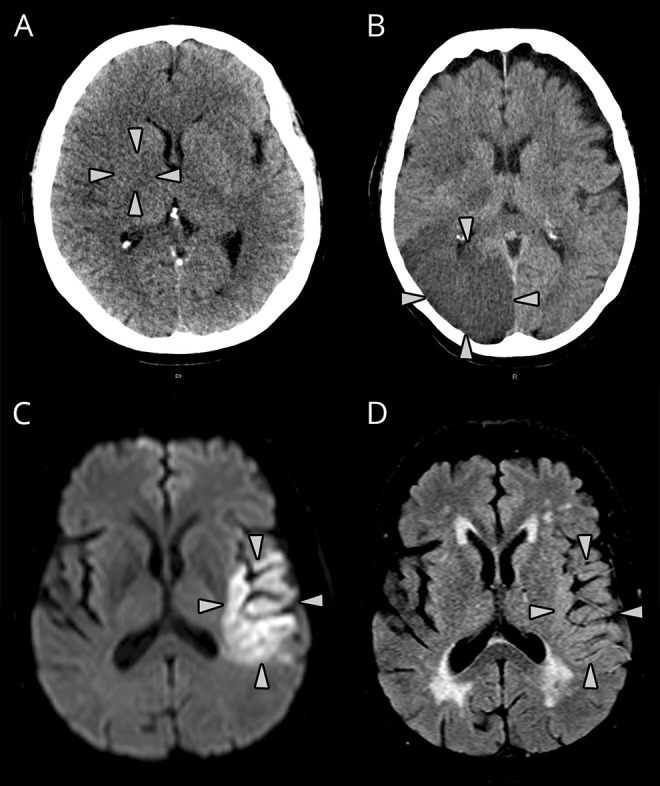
CT and MRI examples of grading of acute ischemic lesion visibility (A) CT lesion visibility grade 1; attenuation of affected gray matter (right lentiform nucleus, arrows) equivalent to normal surrounding white matter. (B) CT lesion visibility grade 2; attenuation of affected right occipital lobe (gray and white matter, arrows) less than normal white matter. (C) Diffusion-weighted and (D) fluid-attenuated inversion recovery (T2-based) imaging demonstrating MRI lesion visibility grade 1; acute lesion is clearly visible in panel C (arrows) but only faintly visible in panel D (arrows).
